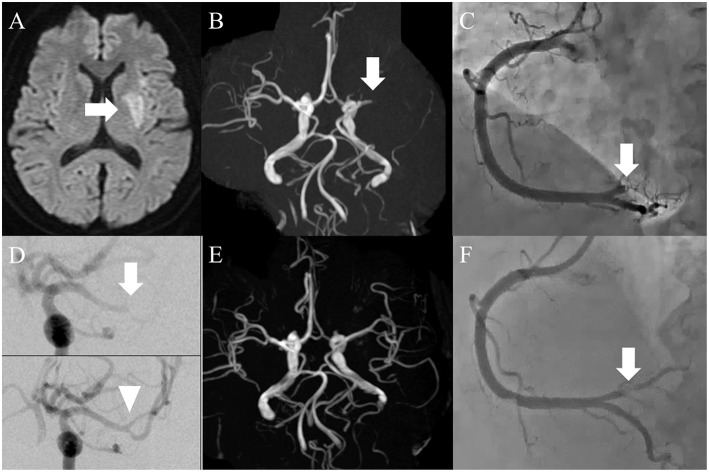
(A) Magnetic resonance imaging on admission day. Diffusion-weighted image showed acute infarction limited to the left basal ganglia (arrow). (B) Magnetic resonance angiography. The left middle cerebral artery (MCA) was occluded in the proximal portion (arrow). (C) Coronary angiography on admission day. The distal portion of the right coronary artery was completely occluded (arrow). (D) Thrombectomy for cerebral artery occlusion (arrow), whereby complete recanalization was achieved (arrowhead). (E) Brain MRA on the second hospital day. The left MCA was recanalized successfully. (F) Coronary angiography post-intervention. Although the stenosis remained (arrow), recanalization was achieved.
